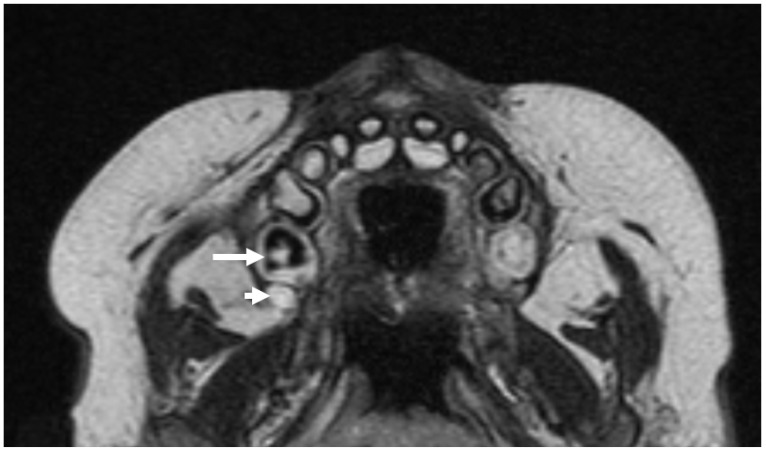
T2-weighted axial MRI through the maxillary dental arch/palate showing a developing primary second molar in the post-appositional phase (long arrow). Note the dark signal corresponding to areas of increased mineralization. Posterior to this tooth germ is a developing first permanent molar tooth germ in the earlier pre-appositional phase without internal areas of dark signal (short arrow).
